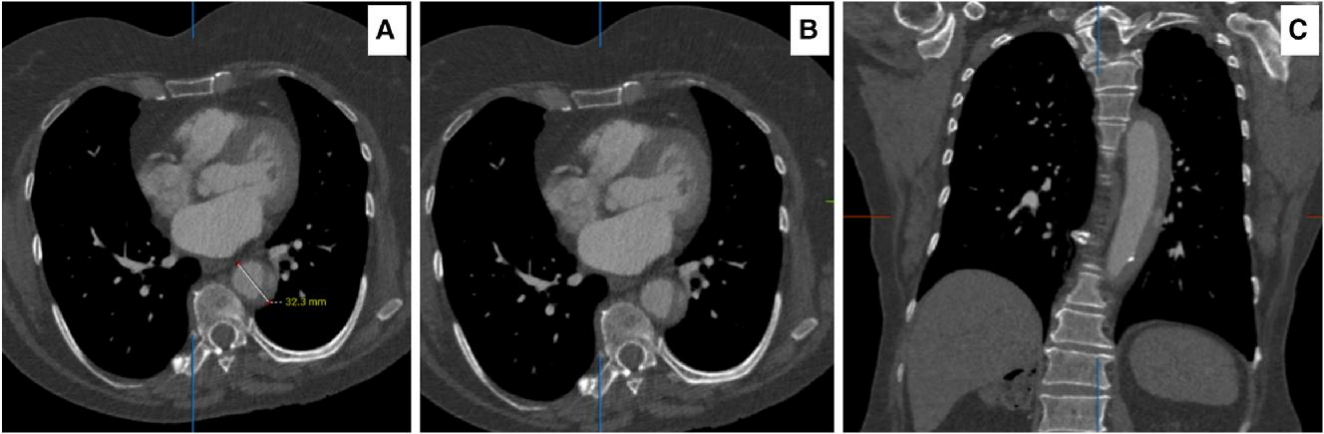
(*A*, *B*) Axial computed tomography angiography 18 h post tyrosine kinase inhibitors and immune checkpoint blockade, acute intra-mural haematoma of 32.3 mm at two levels. (*C*) Sagittal computed tomography angiography demonstrating the length of intra-mural haematoma with the active perforation.
